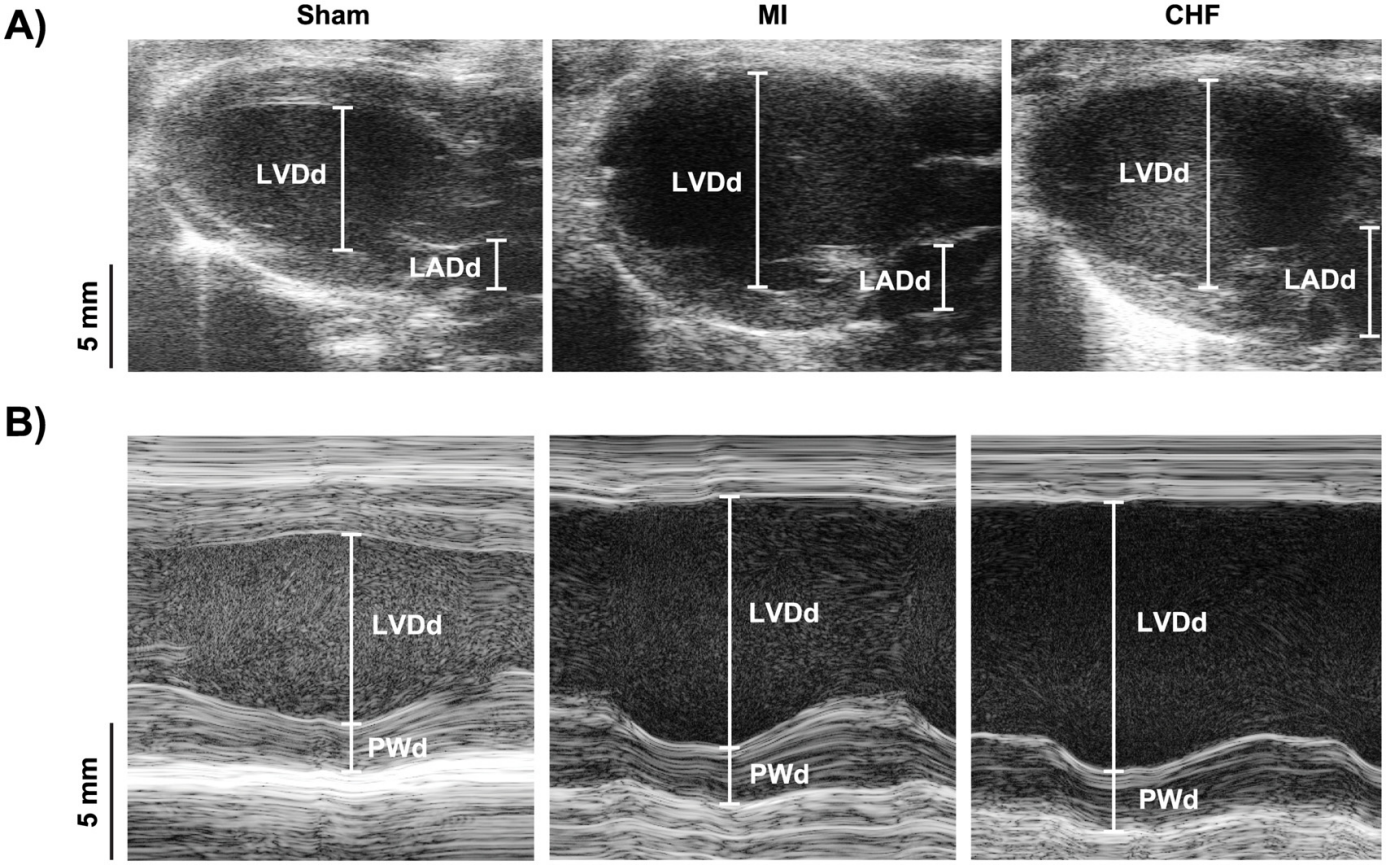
Echocardiographic measurements. Representative echocardiographic parasternal long axis (A) and m-mode (B) images. LVDd, left ventricular diameter in diastole, LADd, left atrial diameter in diastole, PWd, posterior wall thickness in diastole.
